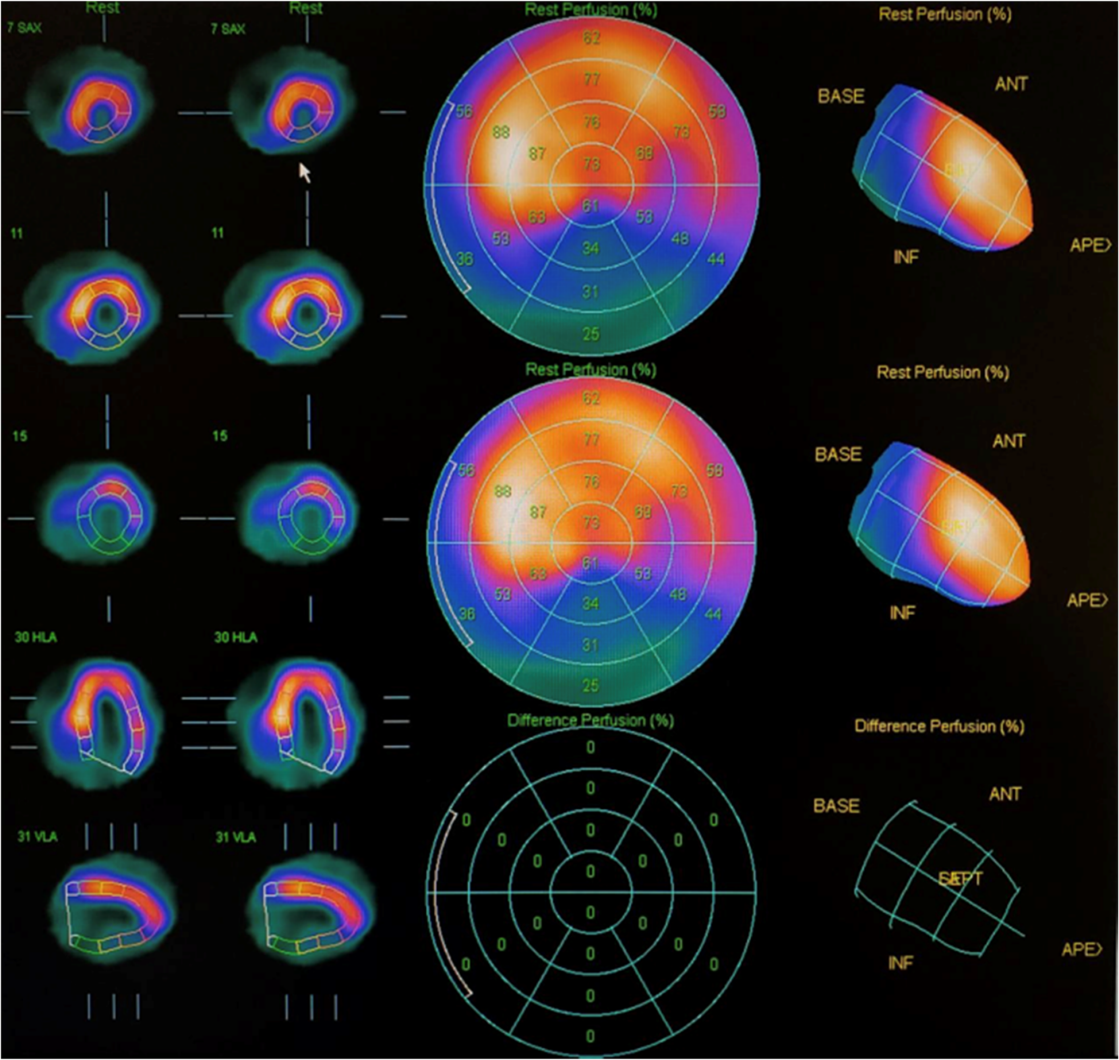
Myocardial perfusion imaging (thallium^201^) by SPECT: showing a myocardial infarction located in inferolateral region, which involved the inferoseptal region; a non-transmural infarction in the apical segment, and transmural in basal and mid segment, without showing viability in delayed redistribution imaging
